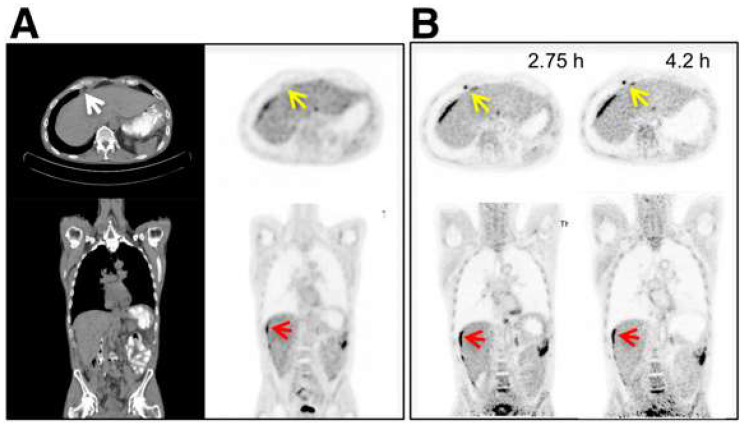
(A) Clinical FDG PET/CT images (transverse and coronal) from subject 5, with metastatic colon cancer, acquired with standard clinical protocol (SAFOV). (B) PennPET (LAFOV) image acquired 2.75 and 4.2 h after injection (10 min scans). Matched coronal and transverse slices are shown. Red arrows denote perihepatic disease; yellow arrows denote epiphrenic lymph node. Image (partially) taken from Pantel et al. CC BY 4.0 License: http://creativecommons.org/licenses/by/4.0/ [2].
